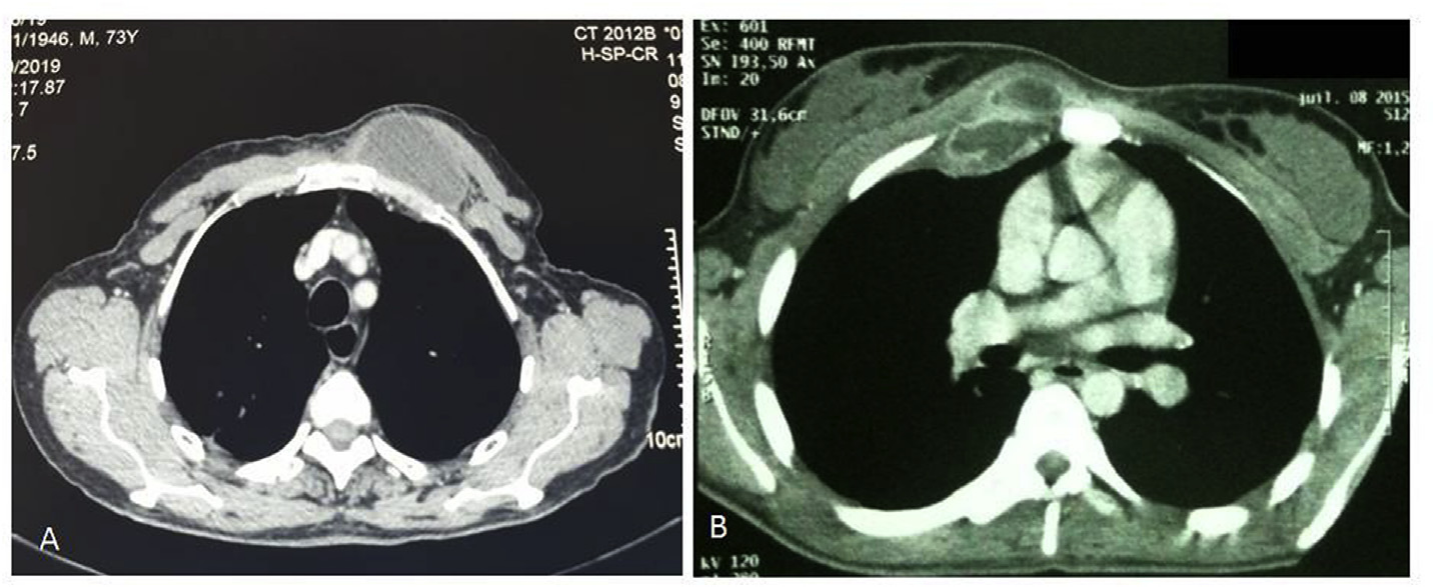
A: Chest computed tomography showing anterior chest wall mass (Patient *no: 16*) B: Chest computed tomography findings showing anterior chest wall abscess with pleural involvement and multilocular form. *(Patient no: 12)*.
